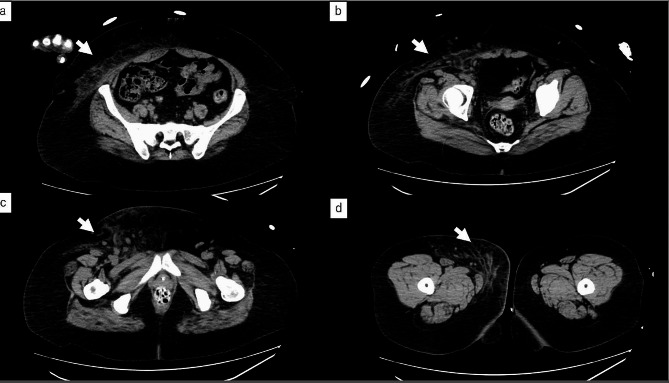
Computed tomography scan of abdominal to femoral region at the first visit. (a): lower abdomen, (b): pelvic, (c): inguinal sutra, (d): femoral region, Increased adipose tissue density was observed from the right side of the abdomen to the right thigh (arrows). No gas images were observed
